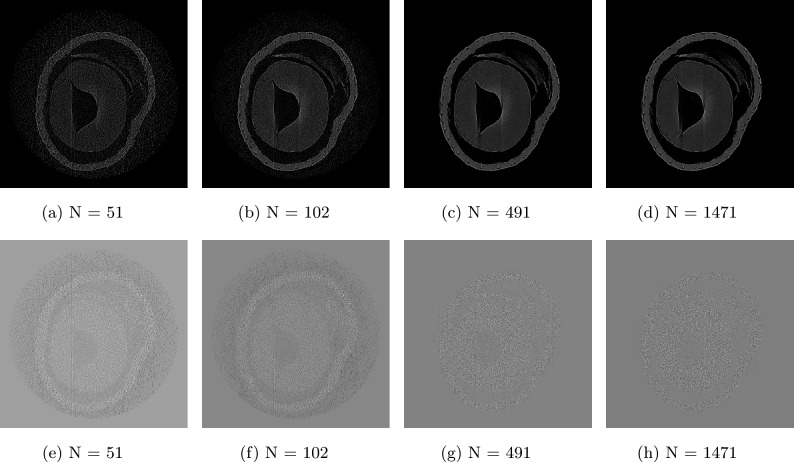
Evaluation of computed tomography (CT) reconstruction quality at slice 262. (a–d) Reconstructions performed using N = 51, 102, 491, and 1471 projections, respectively. (e–h) Corresponding difference images compared to the reference reconstruction using 2942 projections.
